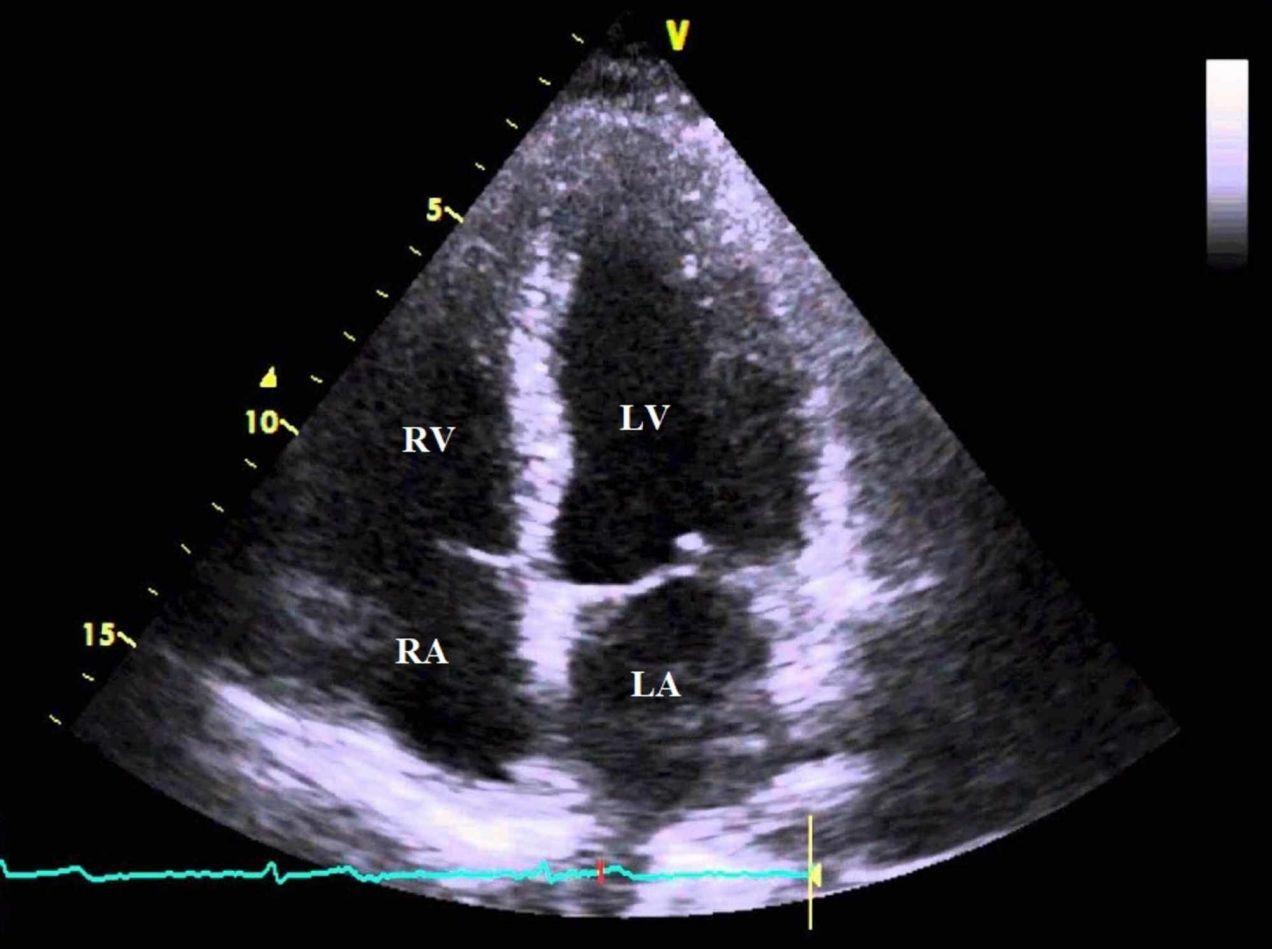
Two-dimensional transthoracic echocardiography (apical 4 chamber view) demonstrating absence of air in the right atrium. RA: right atrium; RV: right ventricle; LA: left atrium; LV: left ventricle
